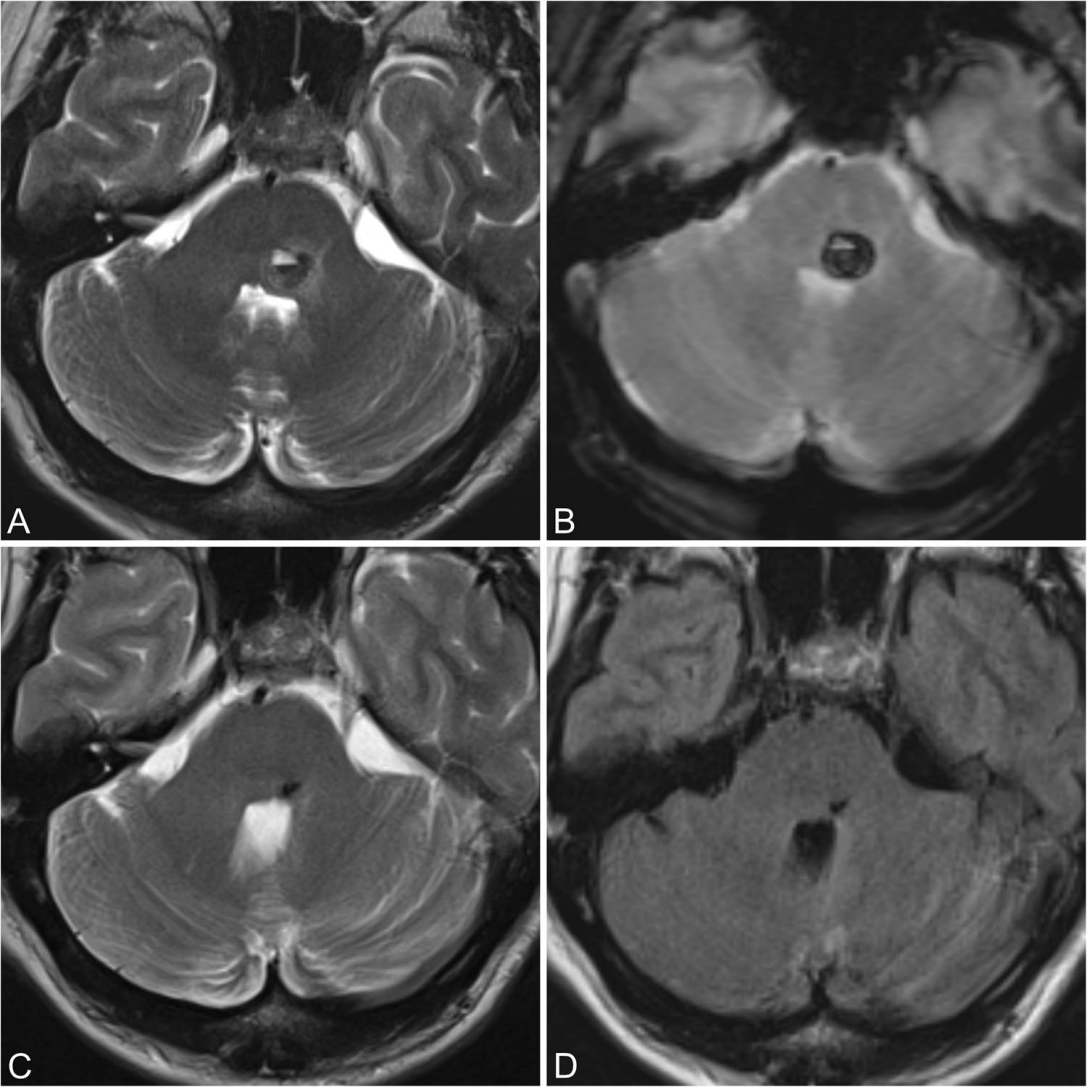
Pontine CM. Preoperative axial T2-weighted image (a) and axial gradient echo image (b). Postoperative axial T2-weighted image (c) and axial T1-weighted image (d). The patient presented with mixed neurological deficits with mRS 2 at the presentation that was better at the follow-up and reported an overall favorable quality of life
